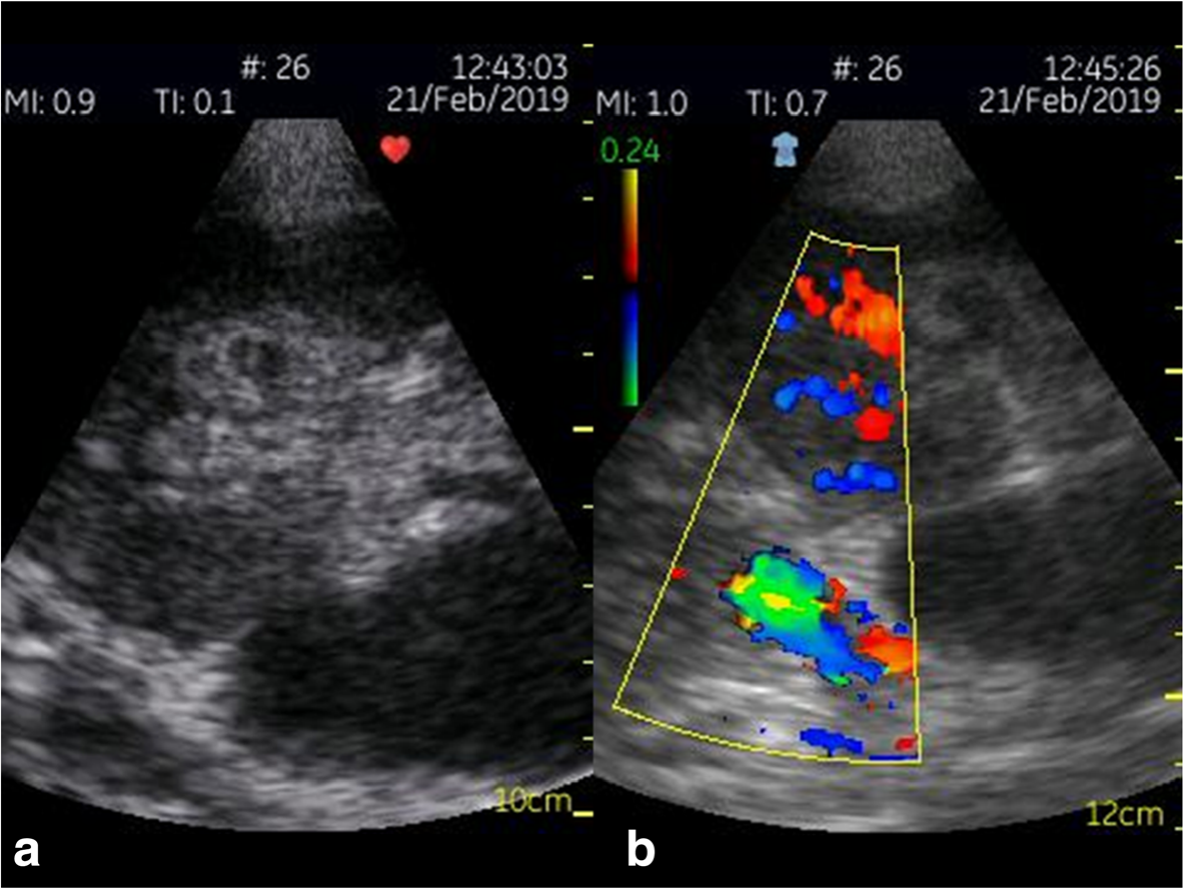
GE Healthcare VScan (phased array transducer): B-scan ultrasound (a) and colour Doppler (b) of a transplant kidney
